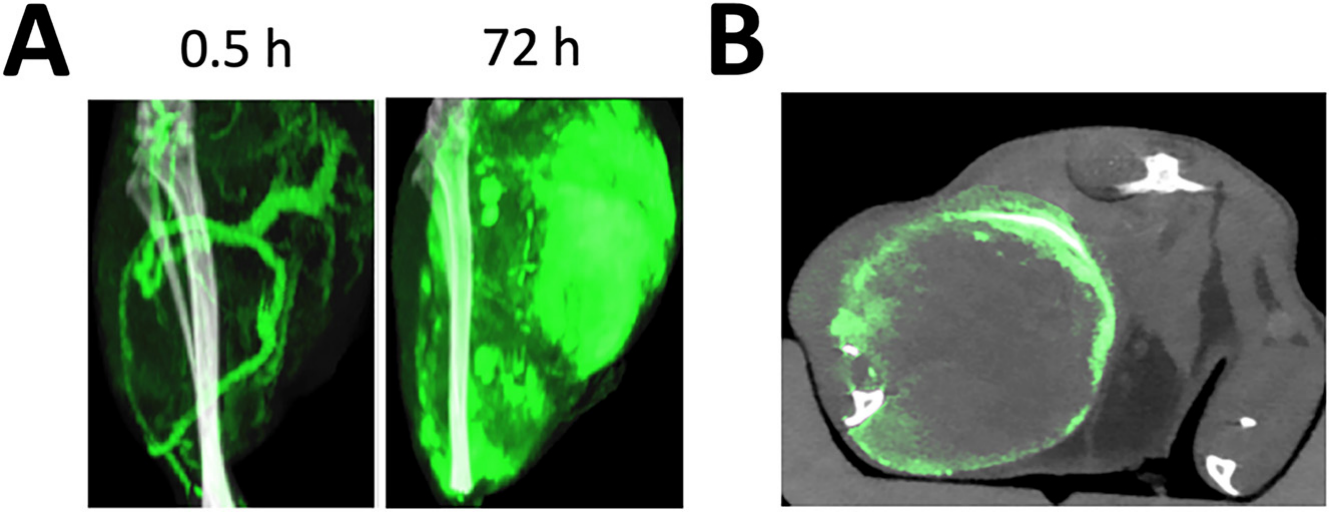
(A) Maximum intensity projection of dual-energy CT images for the tumor in the hind leg 0.5 and 72 h after intravenous injection of GNS. The green color shows GNS concentration in the range of 2–10 mg/ml and the gray color shows tissues windowed from −100 to 5000 HU. (B) Dual-energy CT image for tumor 72 h after GNS intravenous injection. The green color shows GNS in the concentration range from 2.5 to 8 mg/ml and the gray color shows tissues windowed from −500 to 1200 HU. (Adapted from Ref [19]).
